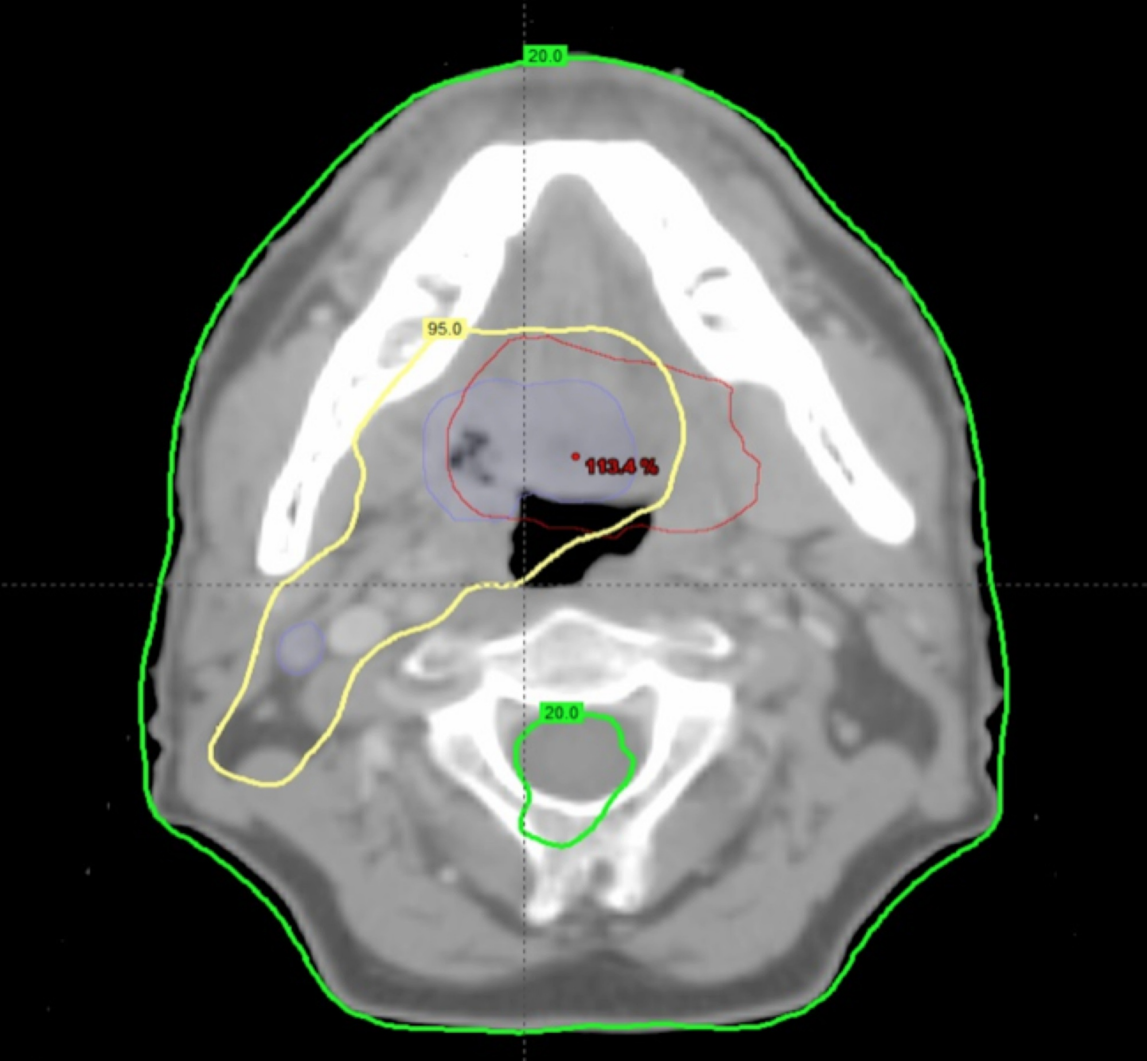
Marginal recurrence T2 N1 carcinoma of base of tongue treated with concurrent chemoradiotherapy; Original gross tumor volume (blue line); Delineation of recurrence (red line) after co-registration with original planning computed tomography; 95% isodose line (yellow); 20% isodose line (green).
